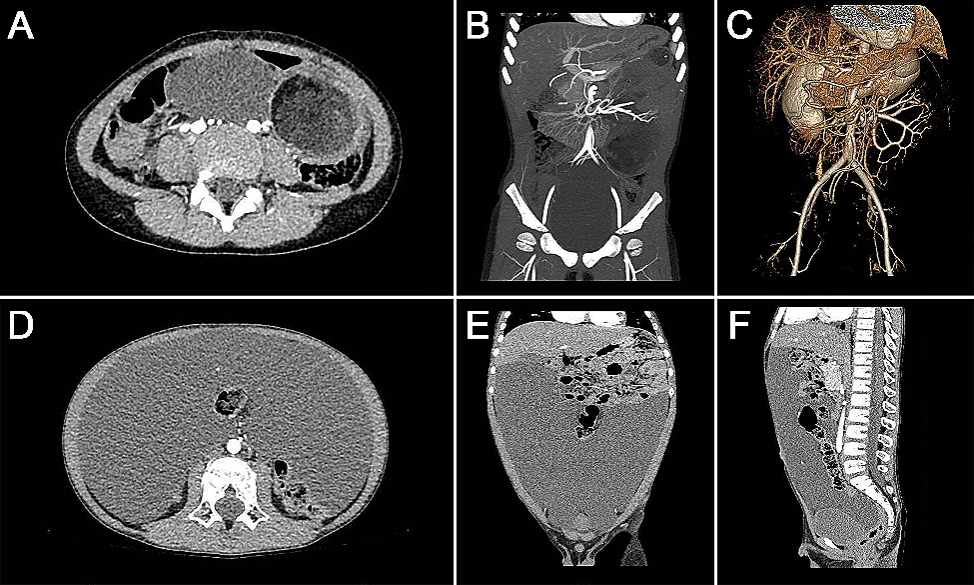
Typical images of ML and OL on abdominal CT. (A-C) Typical images of ML on abdominal CT: A, axial CT image, a mixed cystic mass with wall thickening in the left abdomen; B, CT image reconstruction, the blood supply of the mass was the superior mesenteric artery; C, computed tomography angiography, the superior mesenteric vessels were rotating 360° counterclockwise. (D-F) Typical images of OL on abdominal CT: D, axial CT image, a large amount of low-attenuation intra-abdominal fluid; E, coronal CT image, abdominal mass effect as well as upward bowel displacement; F, sagittal CT image, abdominal mass effect as well as backward bowel displacement
